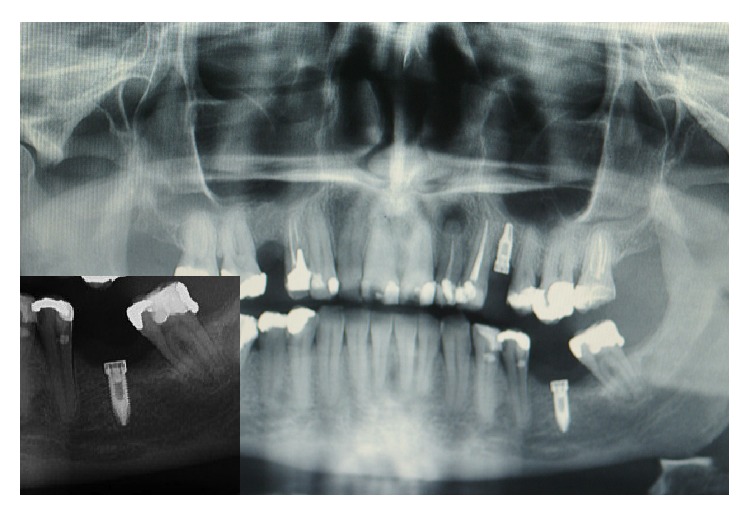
Panoramic radiograph showing the presence of a dental implant in the lower left molar region. Insert: increased magnification focusing on the implant in the region of the lower left molar, showing lack of radiographic features that would be compatible with bone involvement.
